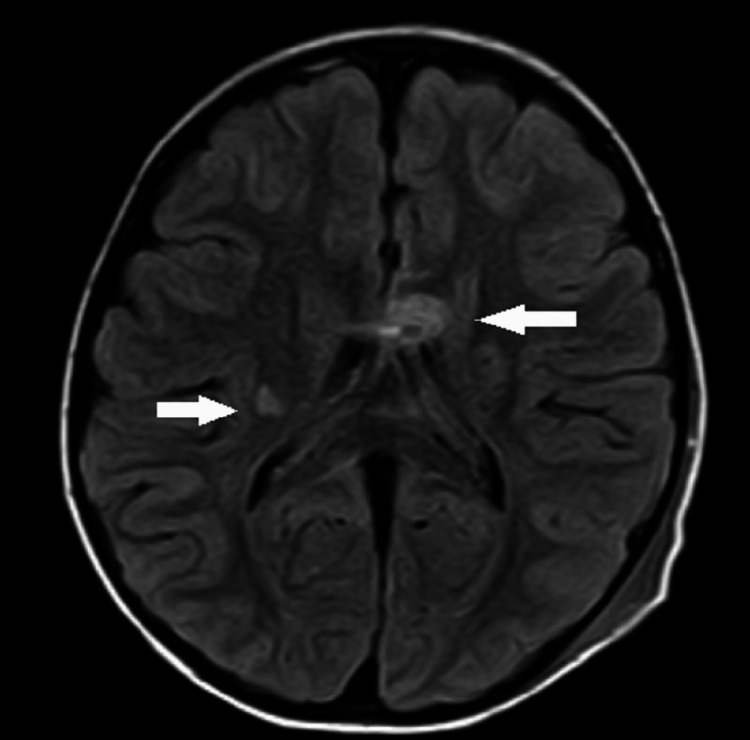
MRI brain axial FLAIR images depicting hyperintense foci involving the right-sided basal ganglia and genu of corpus callosum (white arrows) FLAIR: fluid-attenuated inversion recovery
